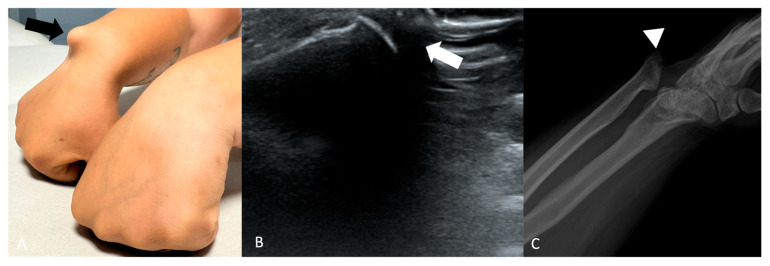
(A) Physical examination of a 27-year-old female shows a bump deformity on the medial side at the level of the radio-ulno-carpal joint (black arrow). (B) US shows the presence of abnormal bone (white arrow). (C) X-rays reveal a severe radioulnar-carpal joint deformity with dorsal ulnar metaepiphysis luxation in fracture outcomes (arrowhead).
